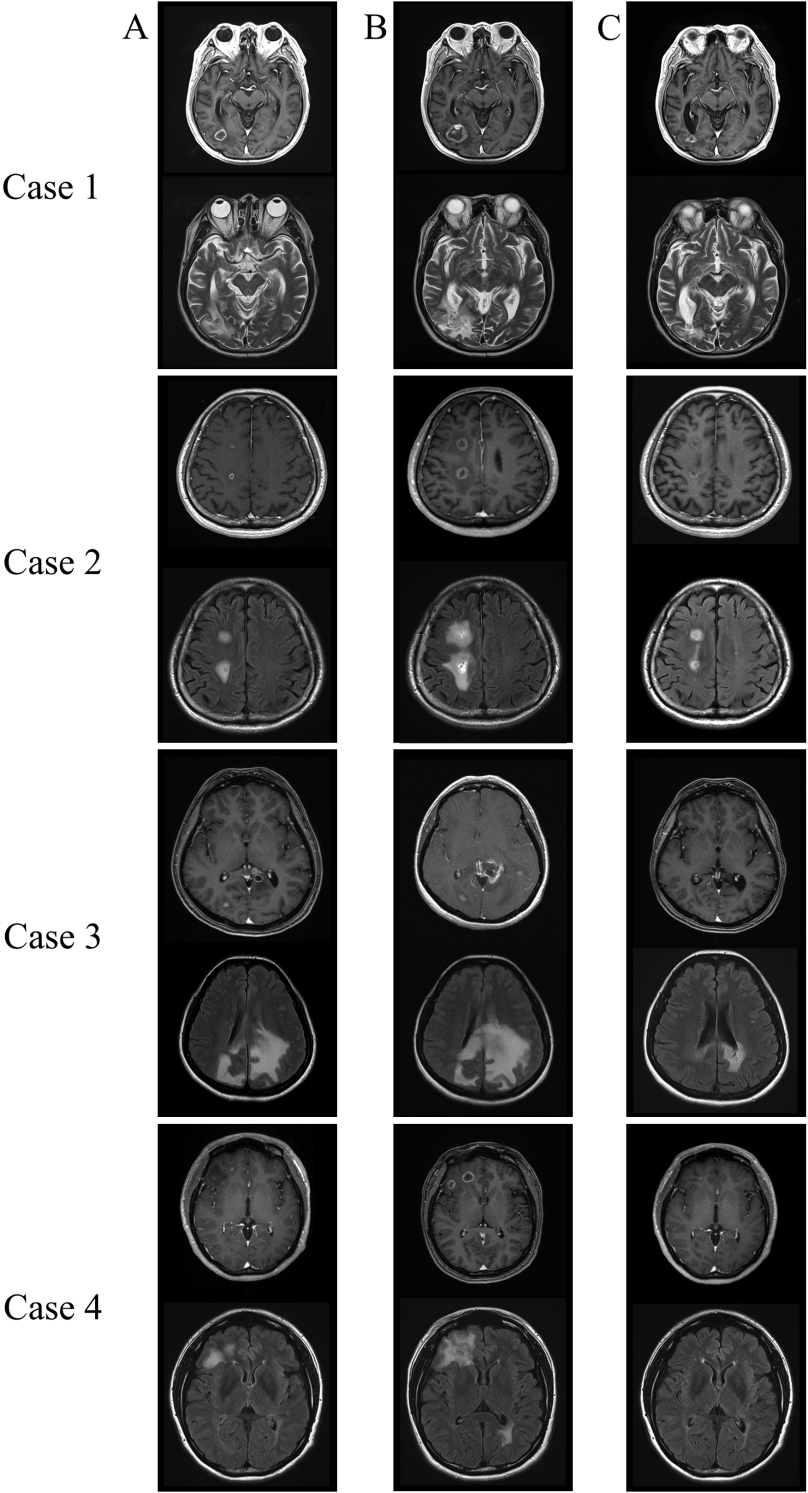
(A, B) Worsening of brain radiation necrosis and brain oedema post‐γ‐knife therapy administration of anaplastic lymphoma kinase‐tyrosine kinase inhibitor (ALK‐TKI). (B, C) Decrease in size of brain radiation necrosis and brain oedema after adding bevacizumab to the treatment regimen. Upper image shows T1‐weighted magnetic resonance imaging (MRI) after gadolinium injection, while the lower image shows T2‐weighted MRI for Case 1. For Cases 2–4, the upper image shows T1‐weighted MRI after gadolinium injection, while the lower image shows fluid‐attenuated inversion recovery (FLAIR) MRI.
